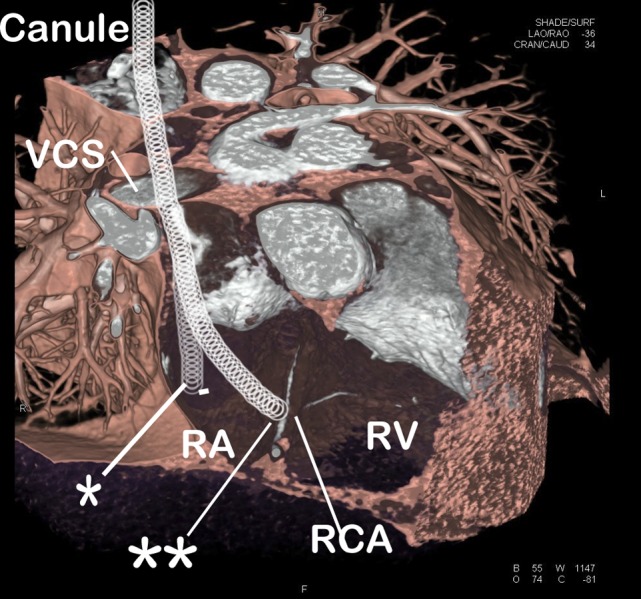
Three-dimensional computed tomography of the right atrium and right ventricle of the heart of a healthy subject (not the described patient). Note the right coronary artery (*RCA*) traveling on the epicardium between the right atrium and right ventricle. The correct cannula position is marked with an *asterisk*, with the tip in the inferior vena cava. Also, the suggested incorrect pathway of the cannula is marked with a *double asterisk* with the tip reaching the tricuspid annulus, compressing the RCA. *RA* right atrium, *RV* right ventricle, *VCS* vena cava superior
